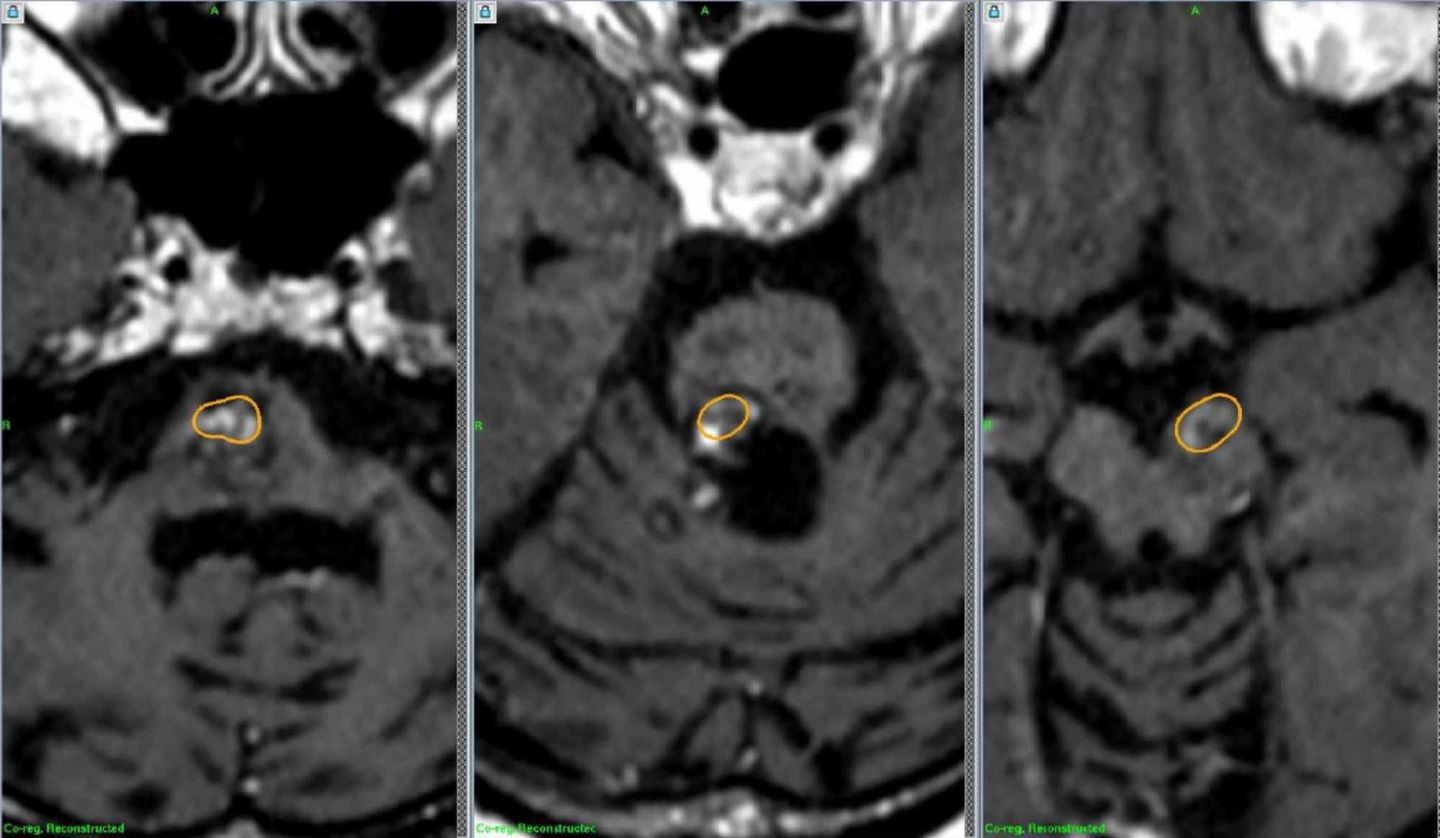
Follow-up MRIs after radiosurgery Coregistrated coronary MRI studies 12 months after radiosurgery showed no remarkable changes of the lesions in the medulla, pons, and midbrain,  when compared with the dose distribution at radiosurgery (encircled).
